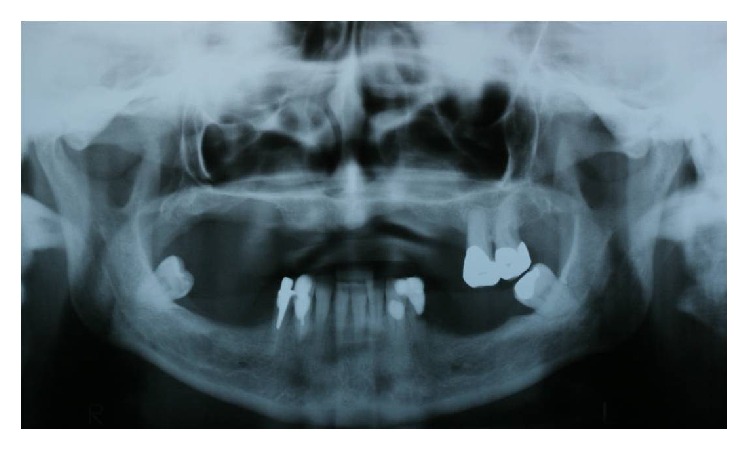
Panoramic radiograph from the initial examination.
